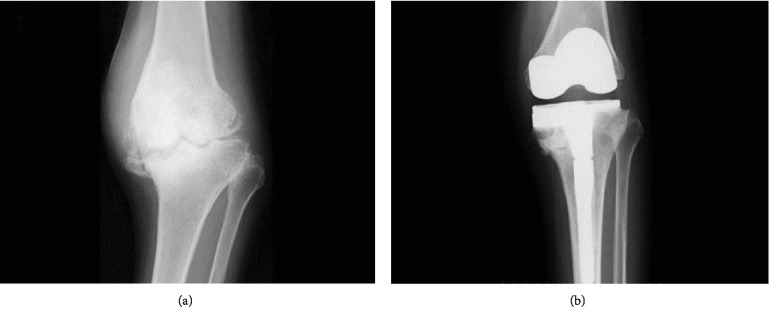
(a) Preoperative radiograph of the left knee showing severe varus. (b) Postoperative radiograph after left TKA.
